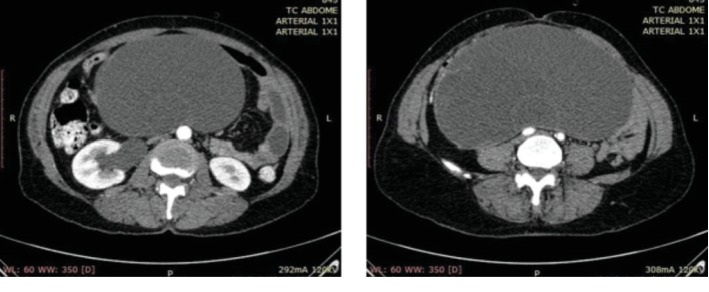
Computed tomography in axial reconstruction.
